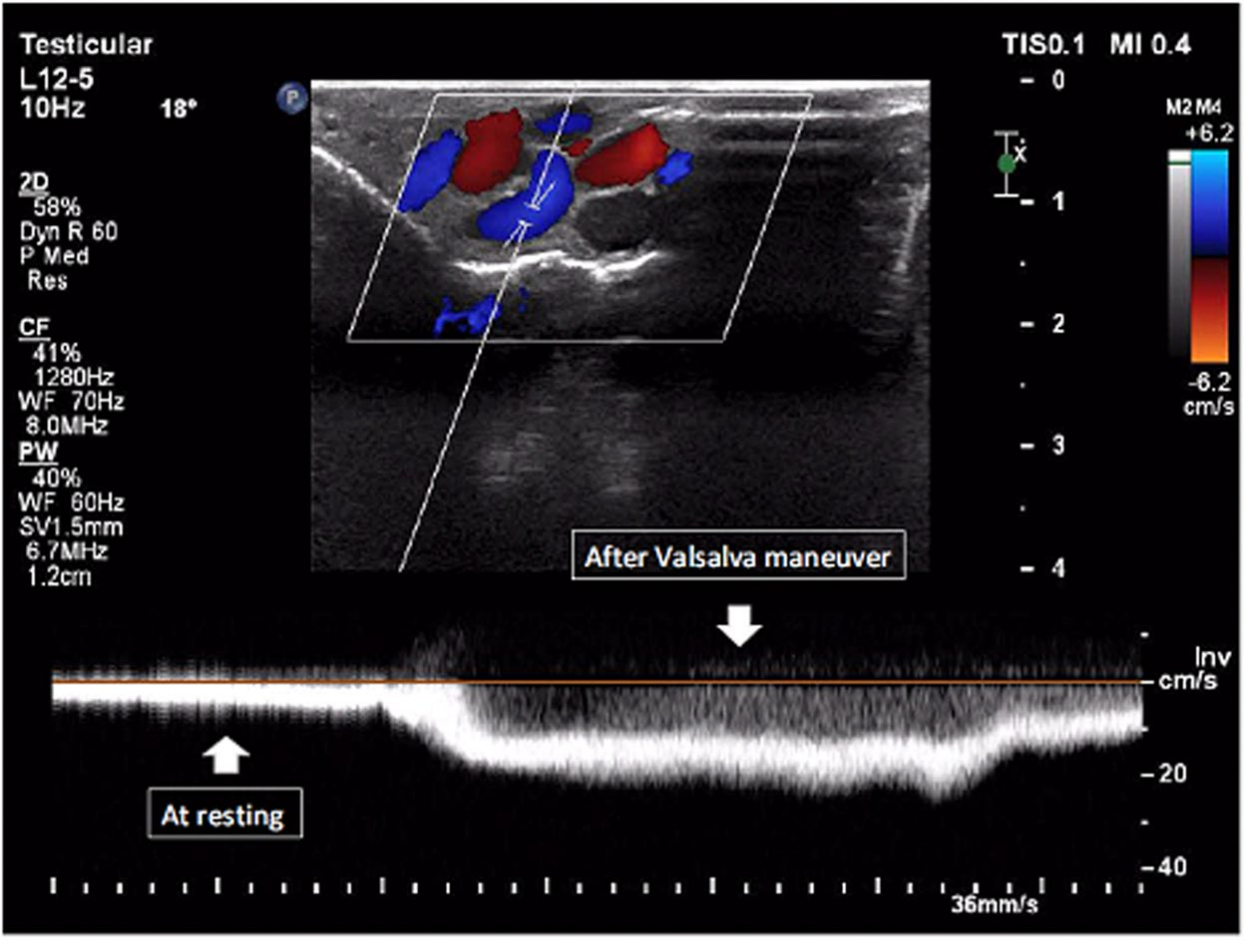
Enhancement pattern reflux: A color (red or blue) was seen during rest, but the same color enhanced during the Valsalva maneuver, and in Doppler spectrum, venous flow increased during the Valsalva maneuver.
